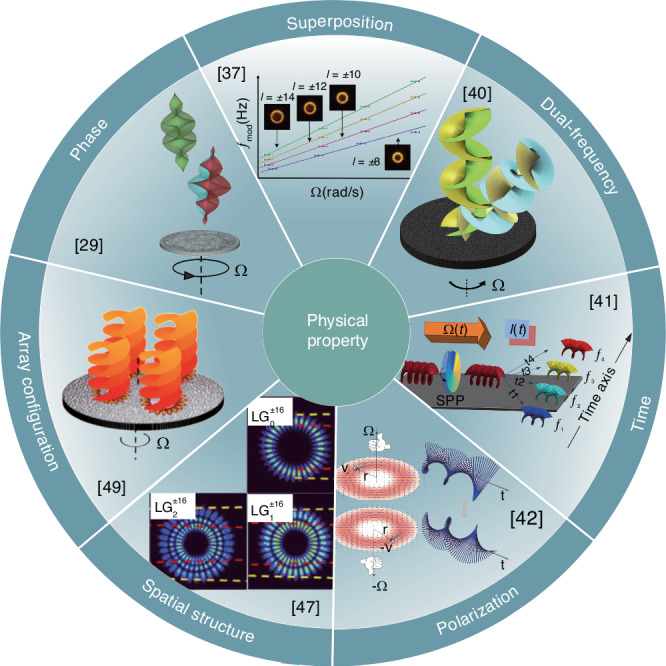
OAM Doppler metrology. Enhancing Doppler metrology with a helical phase of vortex beams, by integrating superposition, dual-frequency, time, polarization, spatial structures, and array configurations, improves signal amplitude, accuracy, and robustness while enabling rotational direction and acceleration analysis. Amplified RDE arises when opposite OAM modes interact with a rotating object. Dual-frequency vortex beams elevate Doppler signals to higher frequencies, reducing noise and enhancing rotational direction analysis. Time-frequency analysis allows dynamic monitoring of angular velocity and acceleration, while polarization and OAM within a vector RDE framework support simultaneous evaluation of velocity and direction. Exploring the spatial structure of probing beams enhances detection accuracy and efficiency for complex radial targets, and an array of superimposed optical vortex beams increases the signal amplitude and robustness against non-coaxial incidence. References given in the insets
